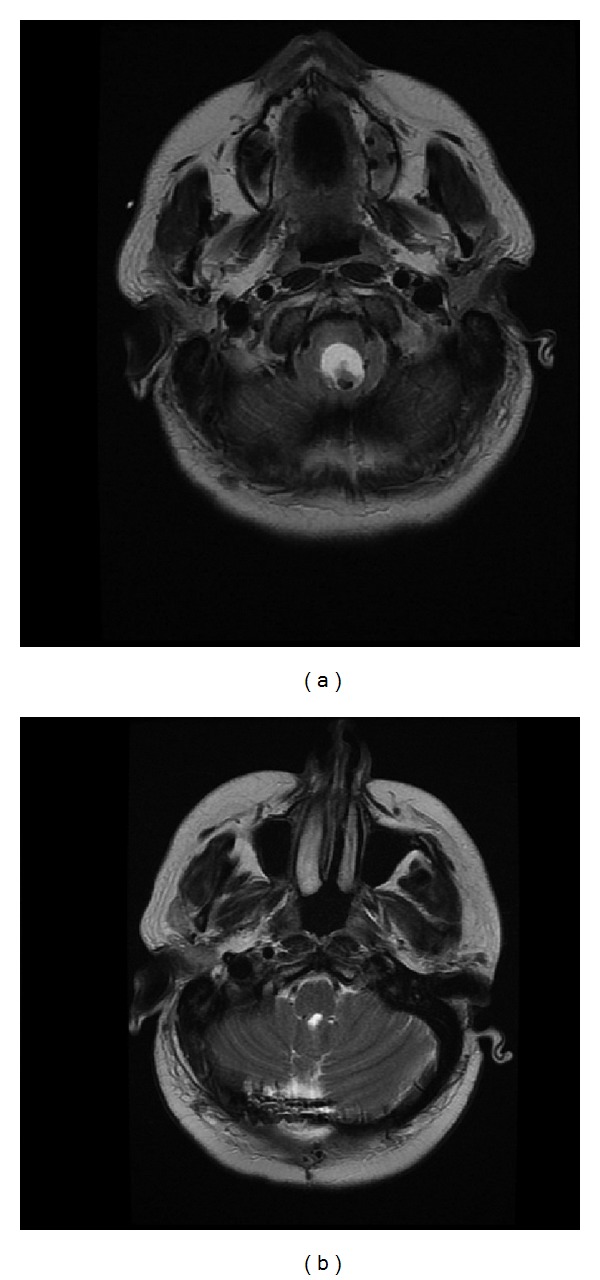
(a) Preoperative T_2_-weighted MRI with axial view depicting an ellipsoid hyperintense cystic mass, hydrocephalus, and brainstem compression. (b) Postoperative T_2_-weighted MRI with axial view depicting successful resection of mass.
